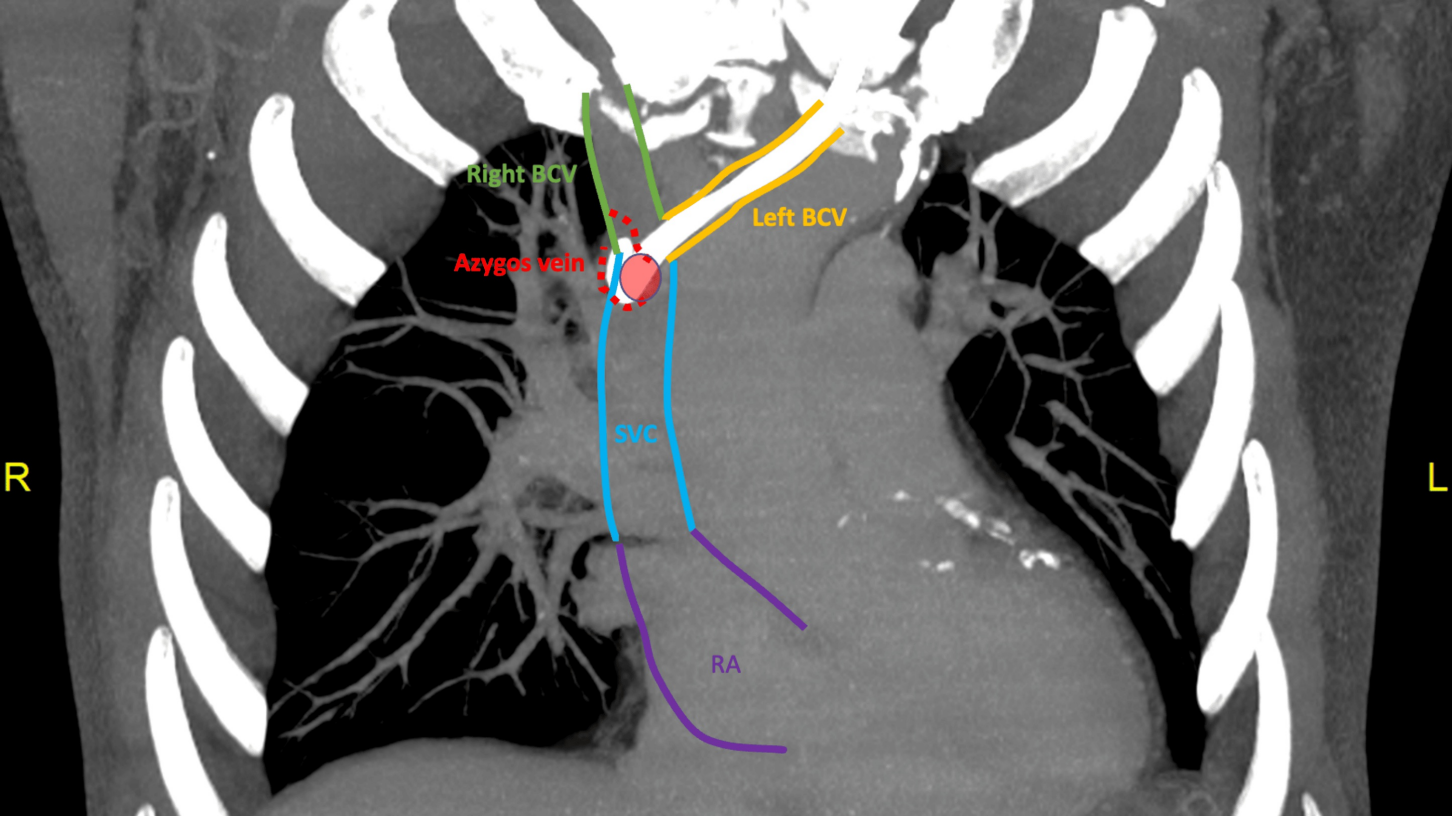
Contrast CT thorax of this case: MIP image in the coronal plane The red circle denotes the azygos opening on the posterior wall of the SVC. The red dotted line shows the course of the azygos vein out of the image plane. The CVC within the left BCV (yellow vessel) meets the SVC (blue vessel) in a more oblique fashion when compared to the right BCV (green vessel). This oblique entry requires the catheter to make a more acute turn to follow the SVC's vertical path; thus, it may inadvertently follow the relatively horizontal course of the azygos vein. The catheter tip within the azygos vein, therefore, gives rise to the fishhook appearance when projected on a frontal radiograph. The purple lines outline the right atrium. MIP: maximum intensity projection, CT: computed tomography, BCV: brachiocephalic vein, SVC: malposition of central venous catheter
